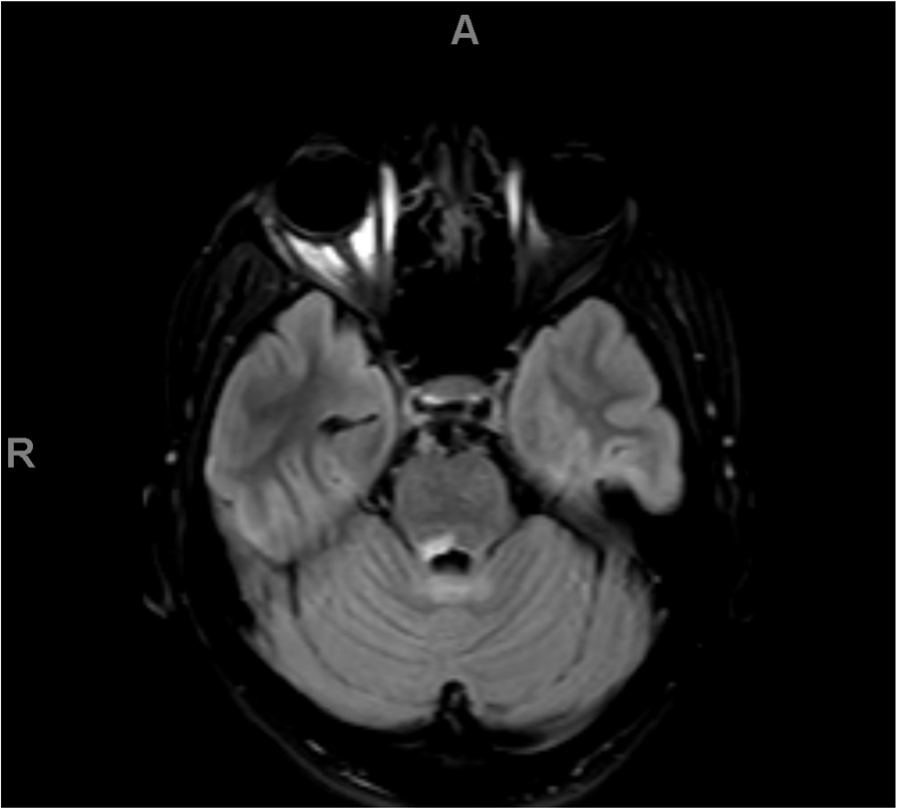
FLAIR axial images demonstrating high signal alterations in the dorsal pons
